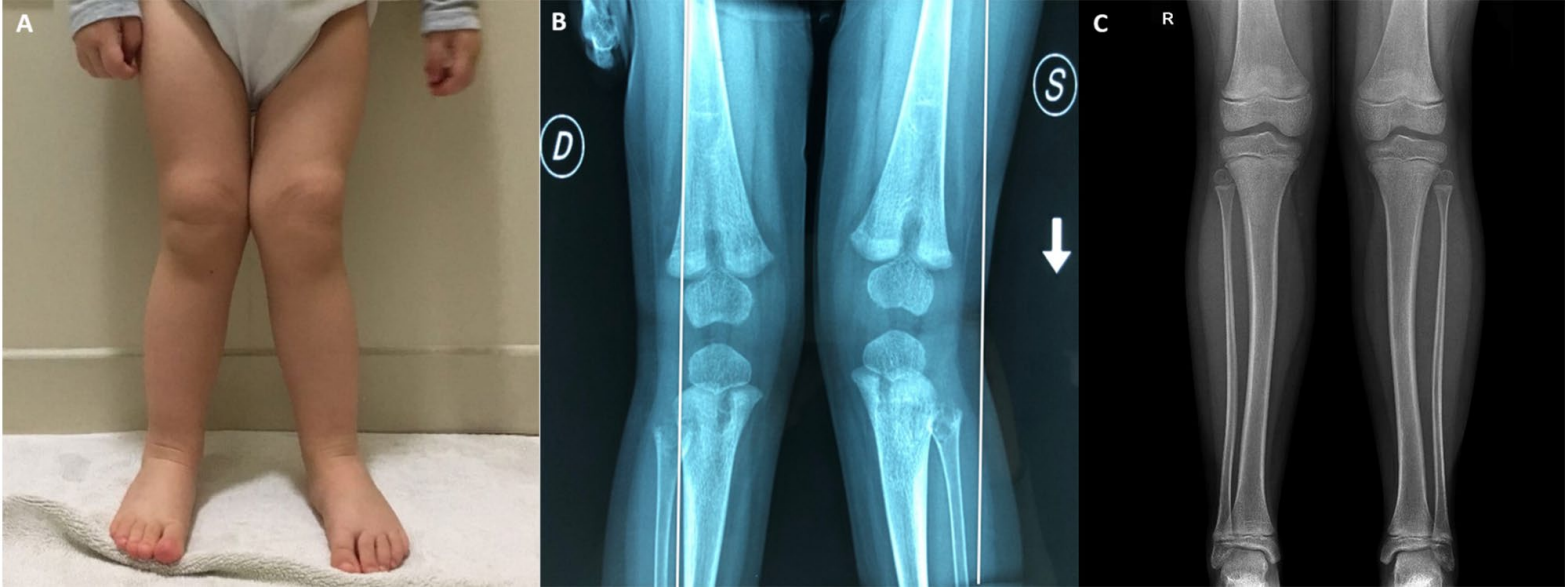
Childhood hypophosphatasia: clinical and radiologic features. (A) A 15-month-old girl presented with limping and genu valgus; (B) At admission, bilateral knee x-ray documented the bilateral presence of typical central metaphyseal radiolucencies, together with various osteolytic areas with sclerotic margins at the peroneal head and at the proximal tibial metaepiphysis, without any interruptions of the adherent cortical; (C) After 6 years of treatment with asfotase alpha, a follow-up radiographic examination showed a “bowing” appearance of the tibial diaphysis without focal morphostructural changes
